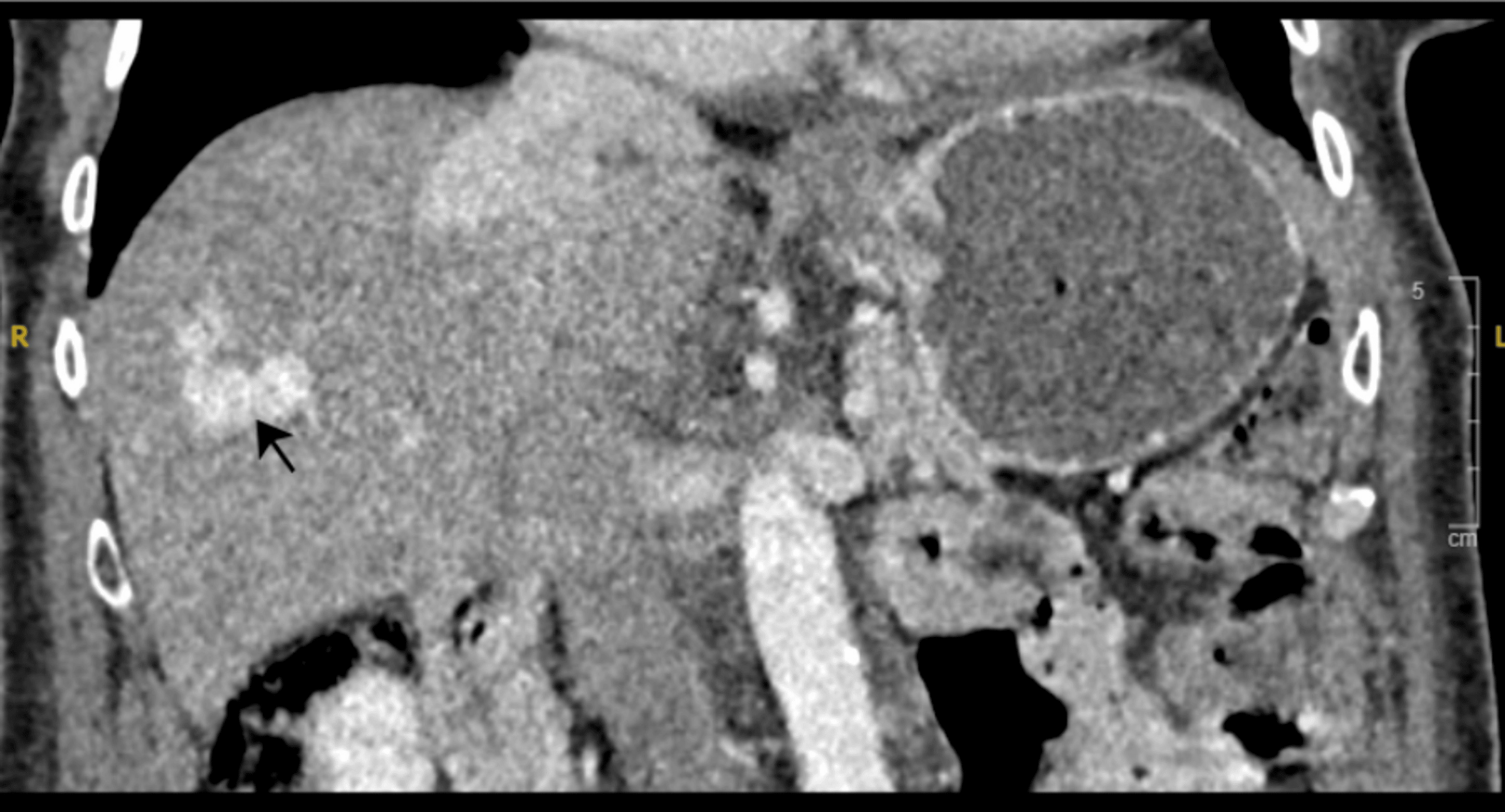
CT scan showing aneurysmal enlargement of shunts CT: computed tomography
